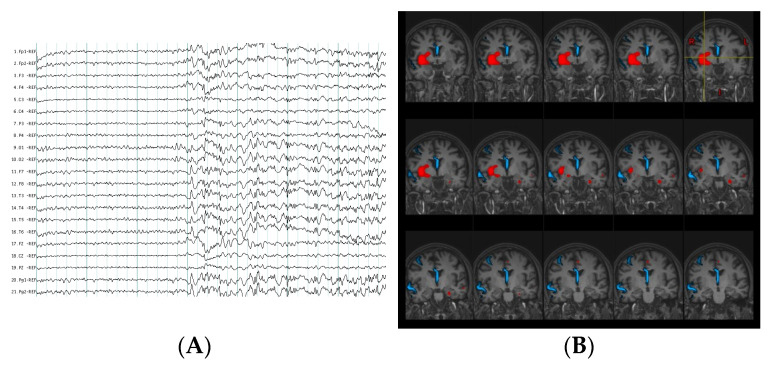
Multimodal evaluation in non-lesional extratemporal epilepsy patient. (A) Ictal scalp electroencephalography (EEG) pattern at seizure onset during habitual non aware focal motor seizures which evolved to bilateral tonic clonic seizures. Visual EEG localization did not show a clear lateralized and localized seizure onset zone. (B) In red, computer- aided subtraction ictal single photon emission-computed tomography (SPECT) co-registered to MRI (SISCOM) of the patient indicated localized areas of hyperperfusion (insula, inferior opercular frontal, putamen, amygdala, and anterior cingulum of the right hemisphere). In blue, estimation of ictal EEG source imaging (ESI) discharges at seizure onset also demonstrated a localize ictal source in this patient (right middle frontal gyrus, right superior temporal and middle line).
